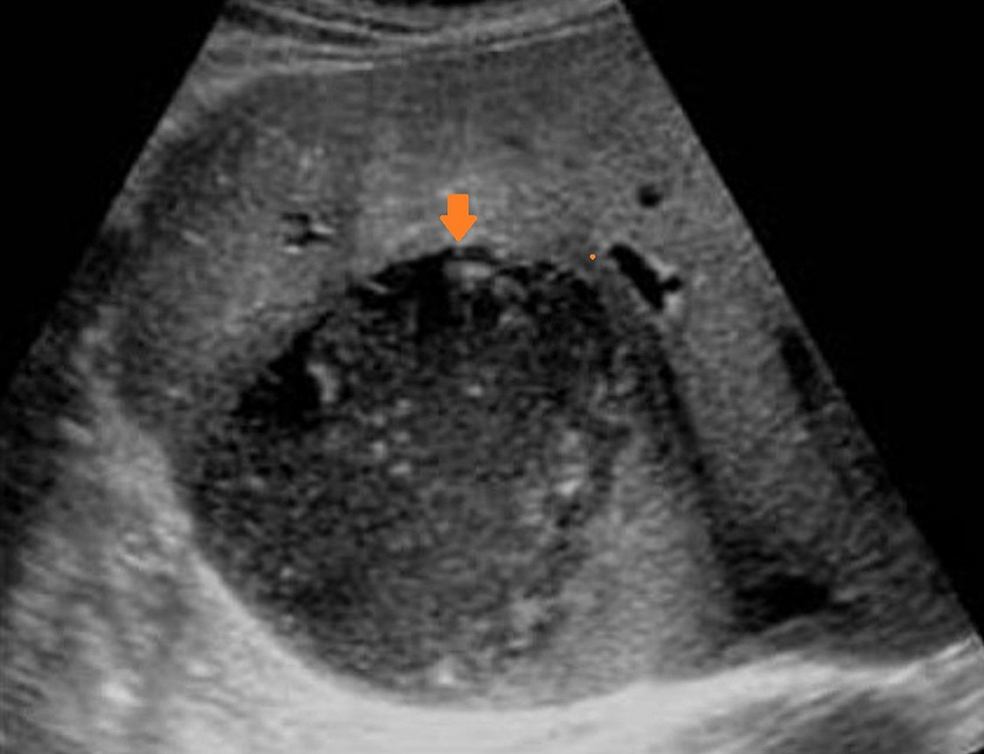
Longitudinal ultrasonography image demonstrating a well-defined collection (arrow) with a hypoechoic wall and internal contents that shows fine low-level echoes consistent with features of amoebic liver abscess. Note the distal acoustic enhancement posterior to the lesion in the right lobe of liver.
